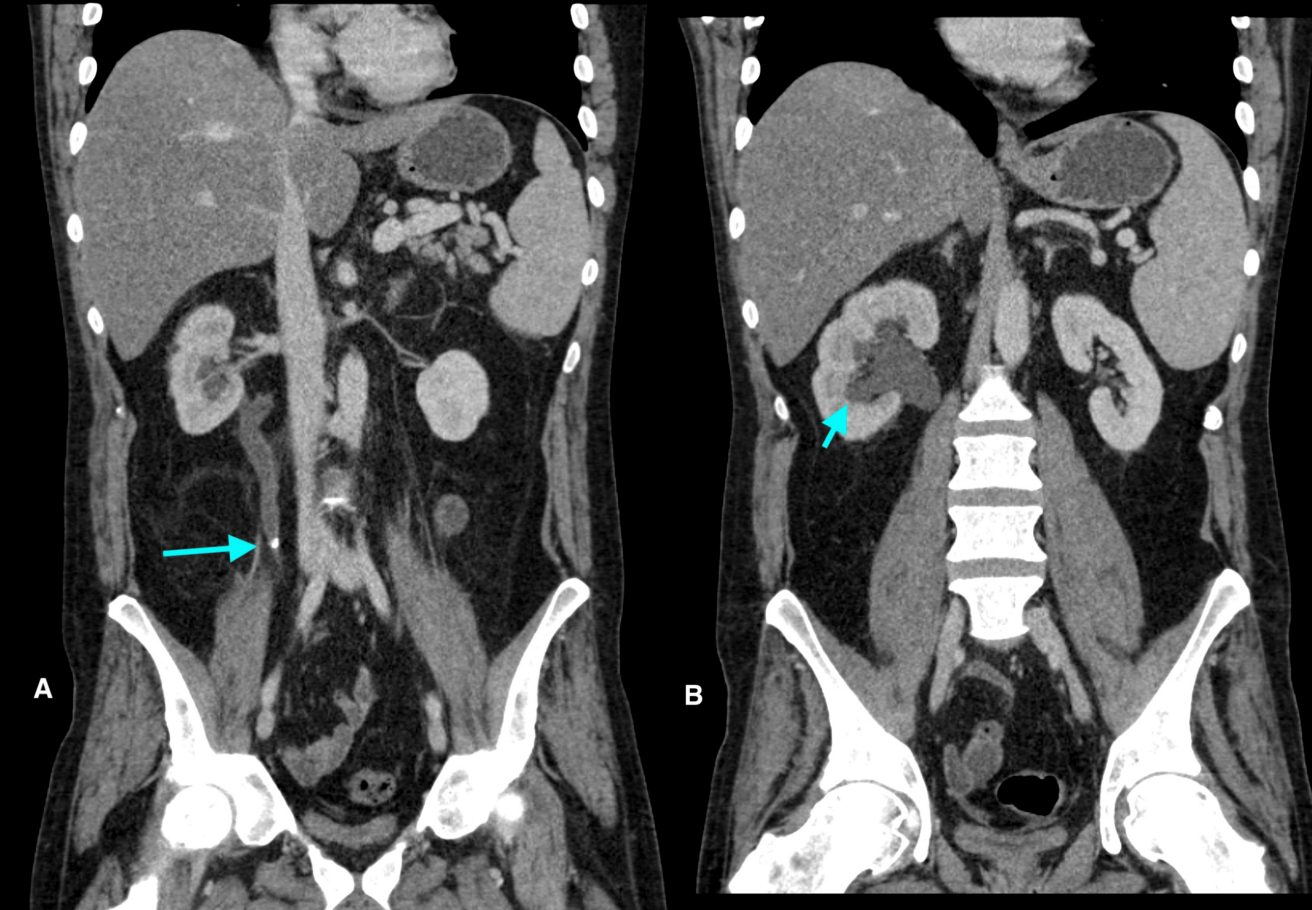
Computed tomography. Initial CT at presentation in 2017 (left) shows an intraureteric right renal calculus (A, long arrow) with upstream moderate hydroureter and hydronephrosis (B, short arrow).
